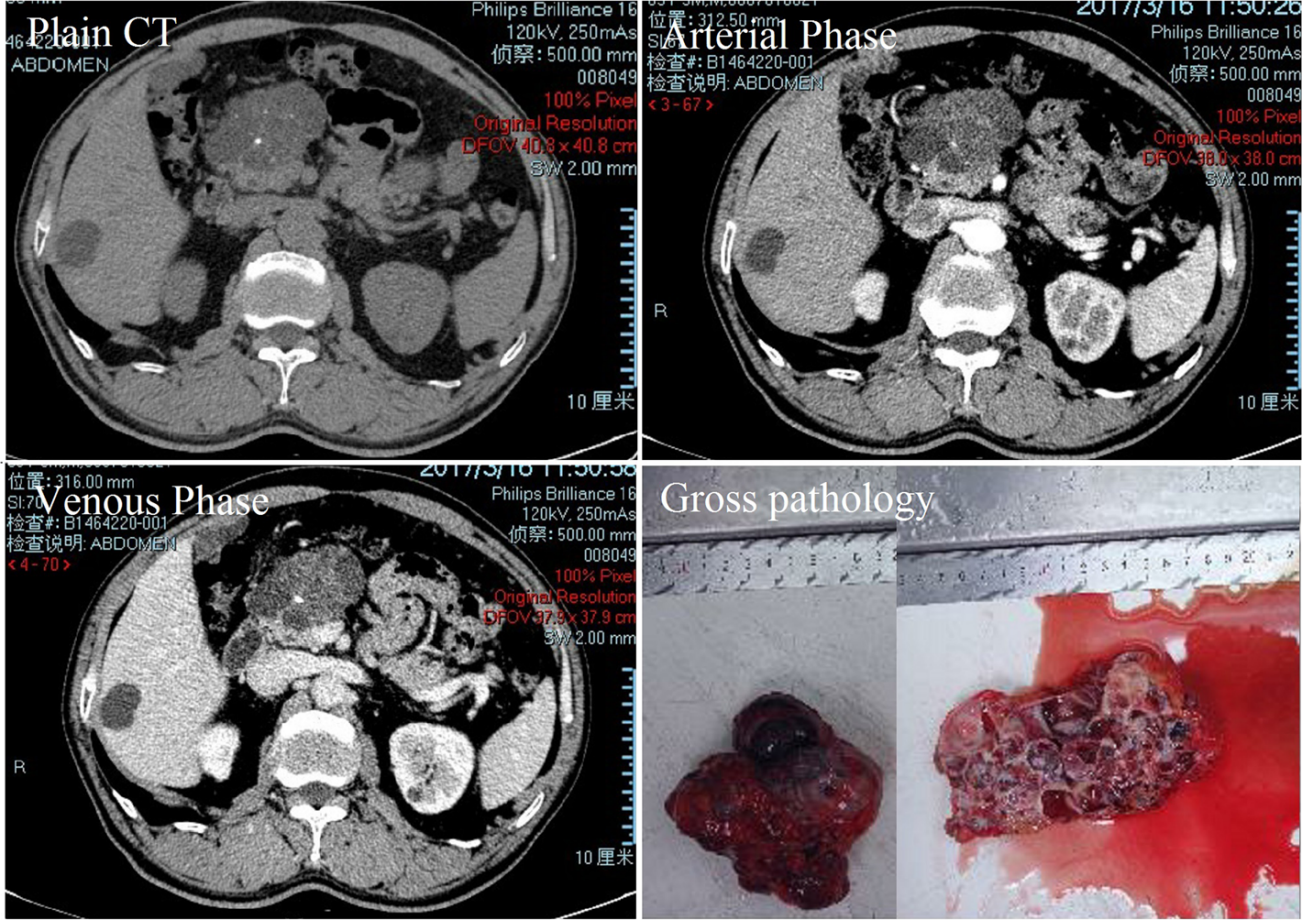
The tumor was a multilocular cyst with septa and fluid-fluid levels. Enhanced scan showing “fast in, slow out”. CT enhancement (arterial phase and venous phase): The CT value was slightly higher than that of plain CT after contrast agent injection at the base of the tumor. Gross pathology showed a cystic lesion with thick septa
